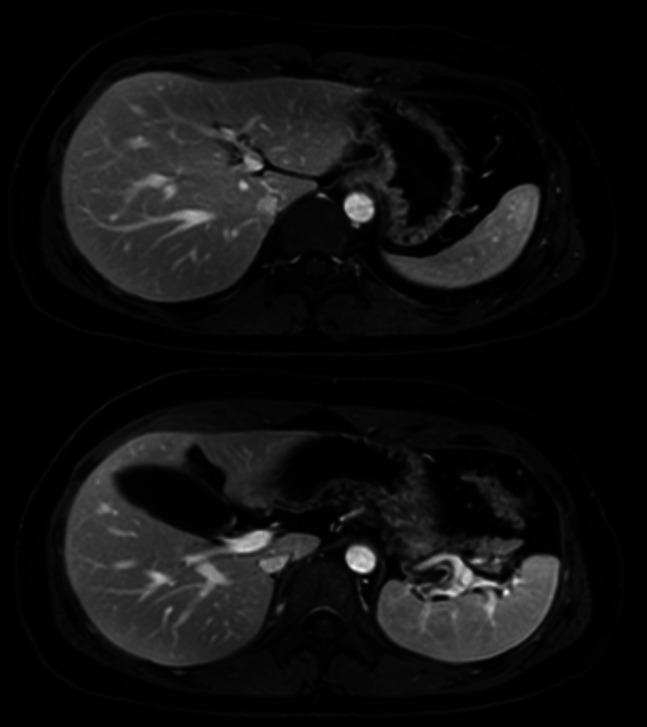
MRI from 2025 demonstrating no signs of cirrhosis, normal sized spleen, and no signs of portal hypertension. Elastography: Calculated mean hepatic stiffness = 1.8 kPa. No findings of steatosis or iron deposition.
